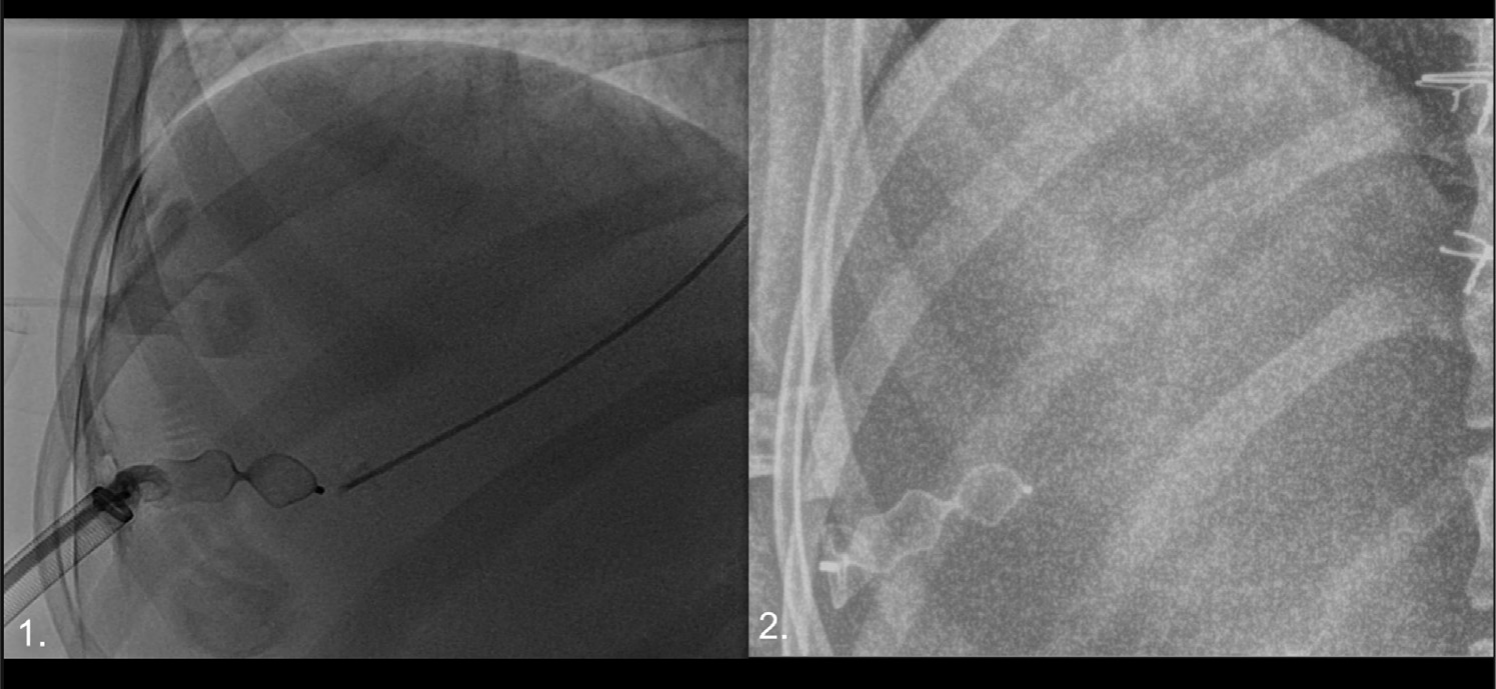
Occlusion of the Hepatic Venous Tract (Left) Fluoroscopic view demonstrating fully deployed 16-mm Amplatzer vascular plug type II. (Right) Abdominal radiograph the morning after the procedure demonstrating fully formed and stable position of the hepatic occlusion device.
